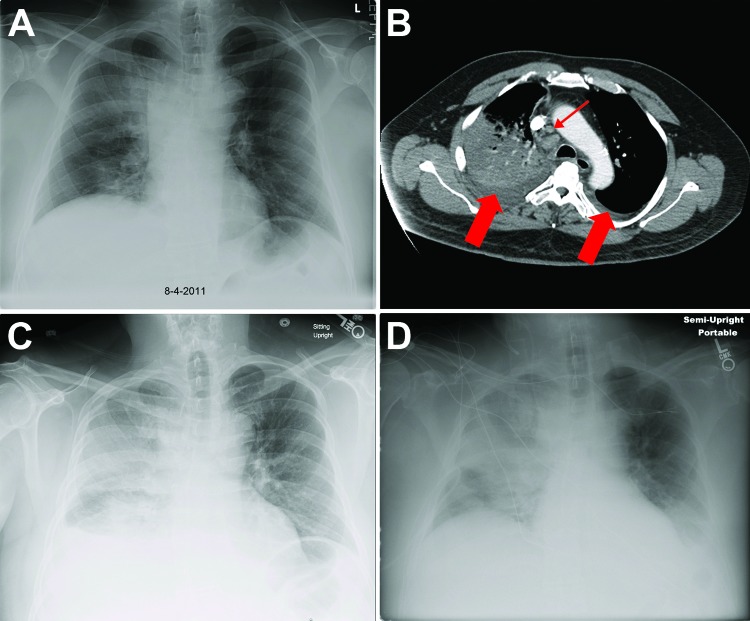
Chest x-ray and computed tomographic scan images for a patient with inhalation anthrax, Minnesota, USA. A) On hospital day 1, the x-ray image revealed a right upper lobe infiltrate and widening of the mediastinum. B) On hospital day 2, computed tomographic scan of the chest with intravenous contrast showed dense consolidation of the right upper lobe, mediastinal adenopathy (small arrow), and bilateral pleural effusions (large arrows). C) By hospital day 4, progressive infiltrates in the right lung were present. D) By day 6, an increasing left pleural effusion was evident.
